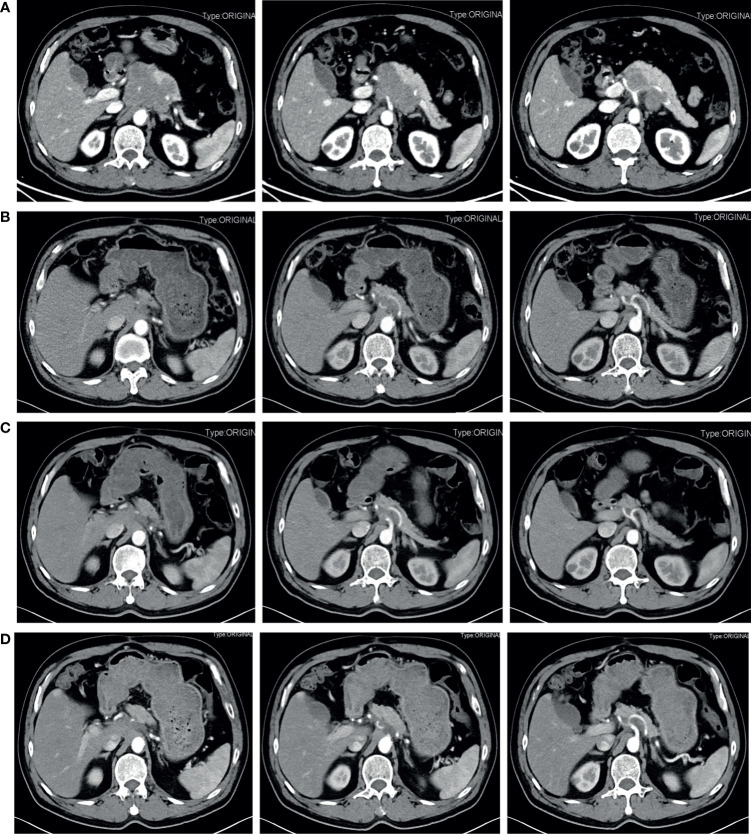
Contrast-enhanced abdominal CT during treatment before the radical surgery. (A) Pretreatment. A retroperitoneal neoplastic lesion measuring about 6.5 cm x 8.3 cm was observed, which was located on the head and neck of the pancreas and manifested a moderate enhancement. The left gastric artery and splenic artery were covered in the tumor, and the common hepatic artery and the celiac trunk were got invaded. (B) 3 months after four courses of etoposide plus cisplatin neoadjuvant chemotherapy. The tumor size was significantly reduced and limited to 2.7 cm x 2.3 cm. (C) 5 months after four courses of etoposide plus cisplatin neoadjuvant chemotherapy. The tumor size was limited to 2.7 cm x 2.3 cm. (D) After six courses of neoadjuvant chemotherapy. The tumor size reached 3.0 cm x 2.2 cm.
